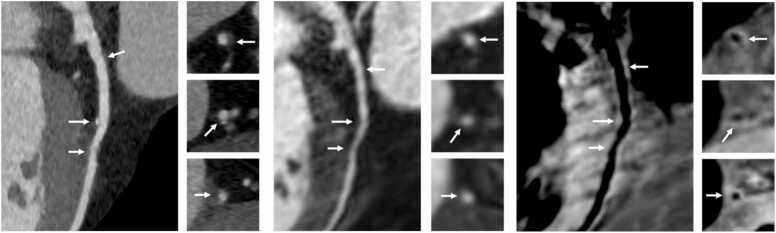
Multiplanar reformat and cross-sectional views of the LAD artery using CCTA and iT2prep-BOOST imaging. Left: CCTA demonstrating multiple plaques (white arrows) in the LAD. Middle: iT2prep-BOOST bright-blood images of the same segments. Right: iT2prep-BOOST black-blood images showing corresponding plaques (white arrows). *LAD* left anterior descending, *CCTA* coronary computed tomography angiography, *BOOST* bright‐blood and black‐blOOd phase SensiTive
